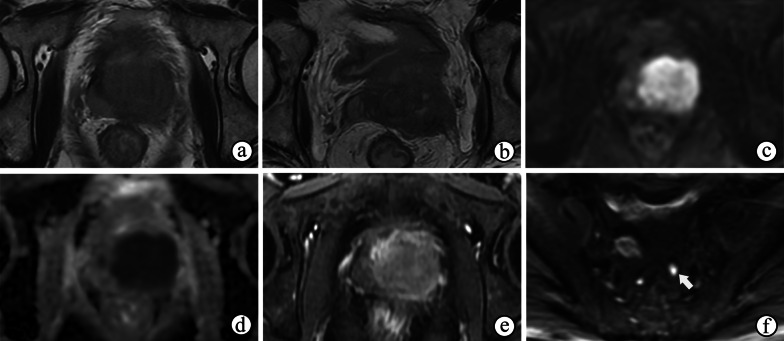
Secondary diffuse large B cell lymphoma involving the prostate in a 66-year-old male with a normal serum prostate-specific antigen level (0.795 ng/mL) and tear-like pain in the lower abdomen for 1 month. a, b T2WI shows a homogeneously isointense mass in the prostate with extraprostatic extension and invasion of the seminal vesicles. c, d DWI at a b-value of 1400 s/mm^2^ and ADC maps show that the mass exhibits observably restricted diffusion. e DCE imaging shows moderate enhancement of the mass. f DWI at a b-value of 800 s/mm^2^ shows slightly enlarged pelvic lymph nodes
